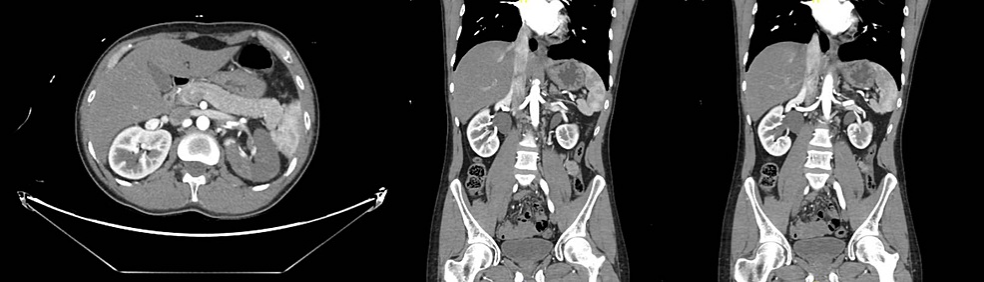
Contrast-enhanced CT scan of the abdomen showing a left renal infarction. CT, computed tomography
